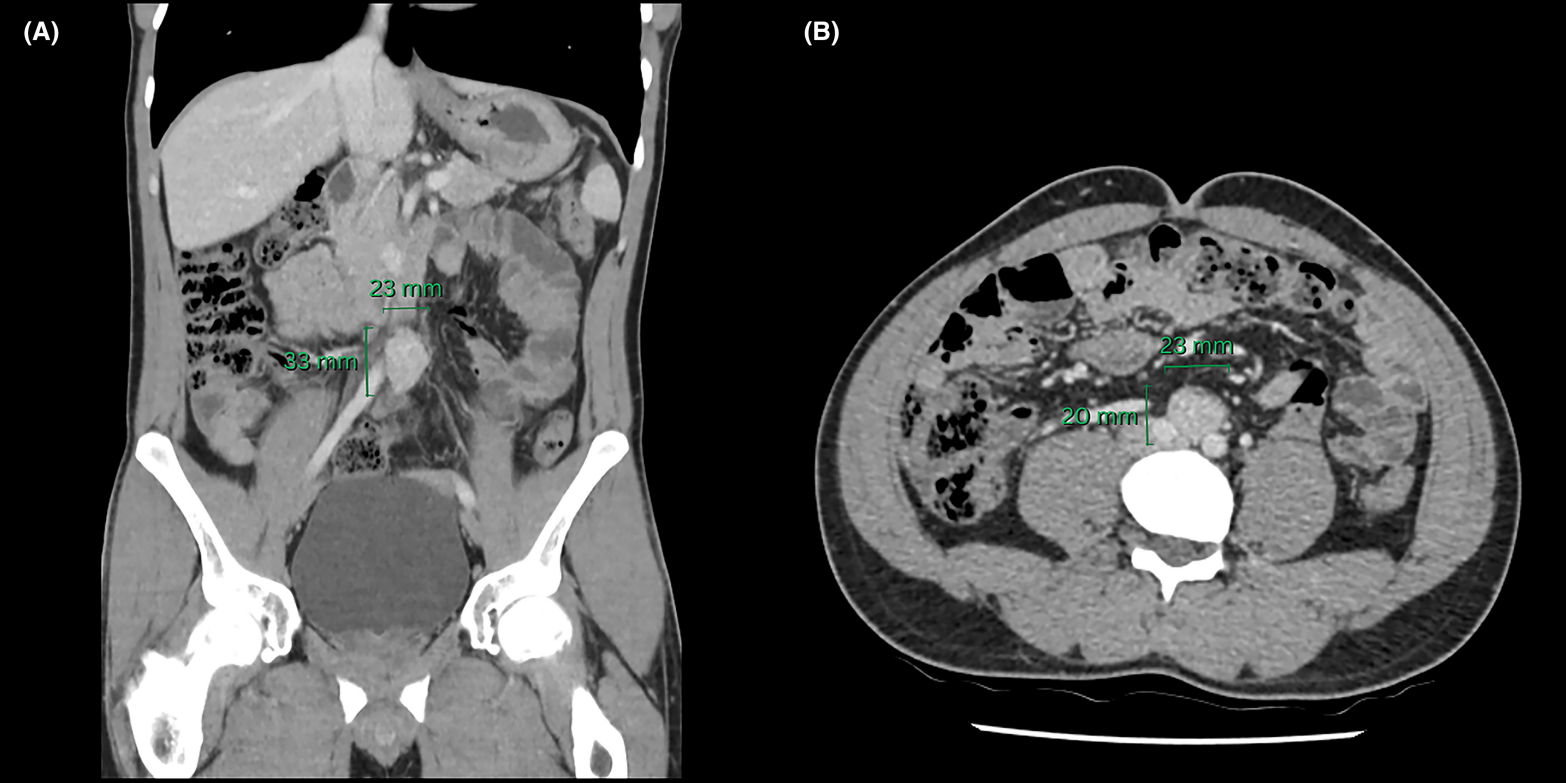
Coronal (A) and axial (B) abdominal CT slices depicting the 33 × 23 × 20 mm enhancing mass near the aortic bifurcation.
